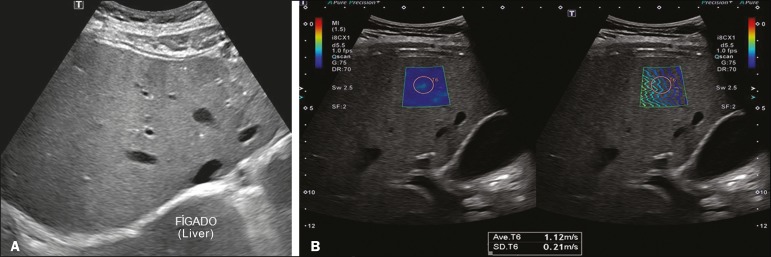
A: Ultrasound examination of a liver with mild steatosis, as characterized by the slightly increased echogenicity of the liver parenchyma. The hepatic vessels and diaphragm are clearly visible. B: A 2D-SWE study showing normal elasticity of the liver parenchyma (1.12 m/s), consistent with a METAVIR stage of F0. The elastography map is homogeneous and predominantly blue, indicating less stiffness. The propagation mode (on the right) indicates normality.
